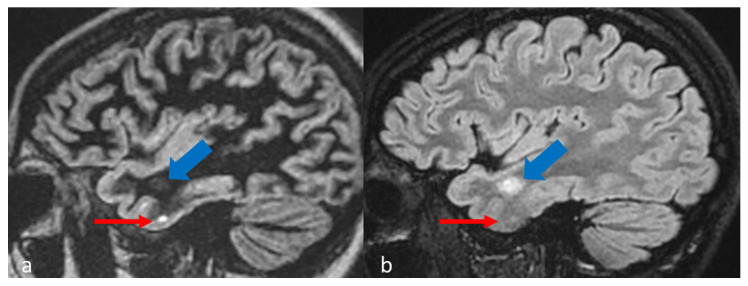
(a) The temporal cortical lesion (red arrow) is well detected in the post-contrast sagittal 3D DIR image; (b) the same lesion is hardly visualized in the post-contrast sagittal 3D-FLAIR. However, the active subcortical adjacent lesion (blue arrow) resulted suppressed in the post-contrast 3D-DIR.
